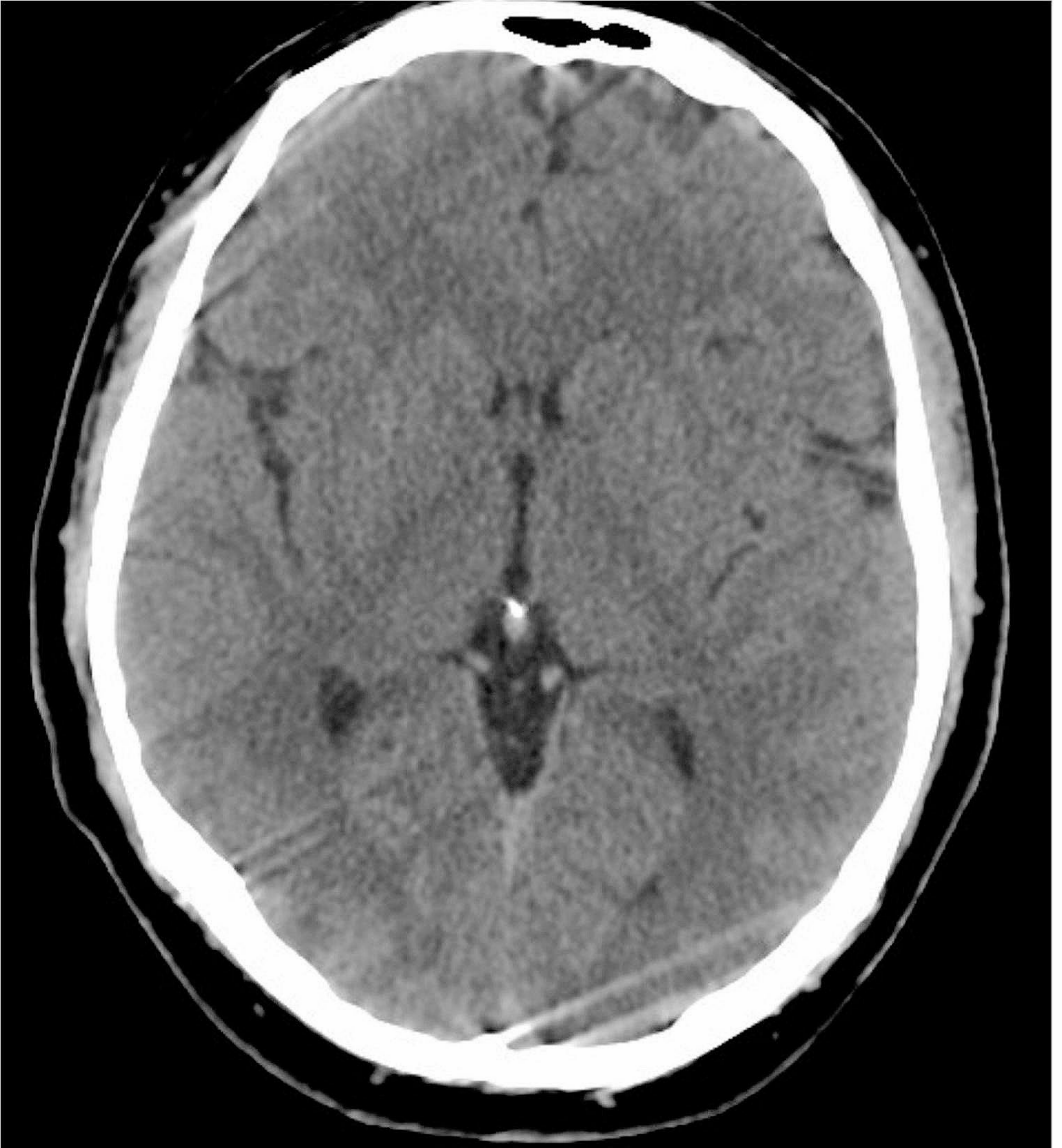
Computed Tomography (TC) Brain
